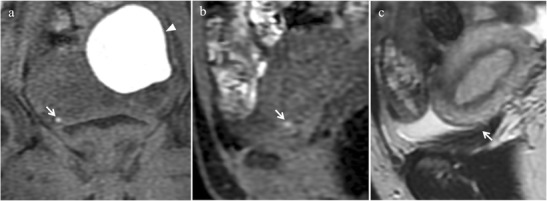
Bladder endometriosis with extrinsic involvement in a 21-year-old woman with left endometrioma and other endometriotic implants involving the left fallopian tube, the uterine serosa and the right uterosacral ligament. (a) Coronal and (b) sagittal fat-suppressed T1-weighted images demonstrate one spot of high signal intensity on the serosal surface of the bladder representing a small peritoneal implant with hemorrhagic content (white arrows). Note the endometrioma in the left ovary (white arrowhead in a). On (c) sagittal T2-weighted image the implant (white arrow) is hardly detectable because it is partly masked by the hypointense signal of the bladder wall
